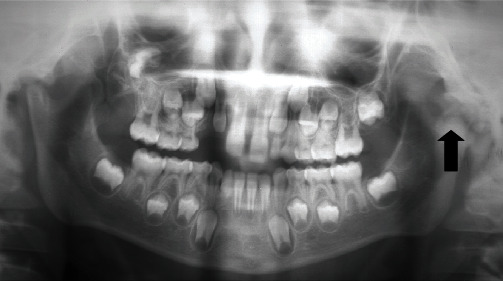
Panoramic radiograph showing ankylotic mass on the left condyle blurring the joint space (black arrow).
